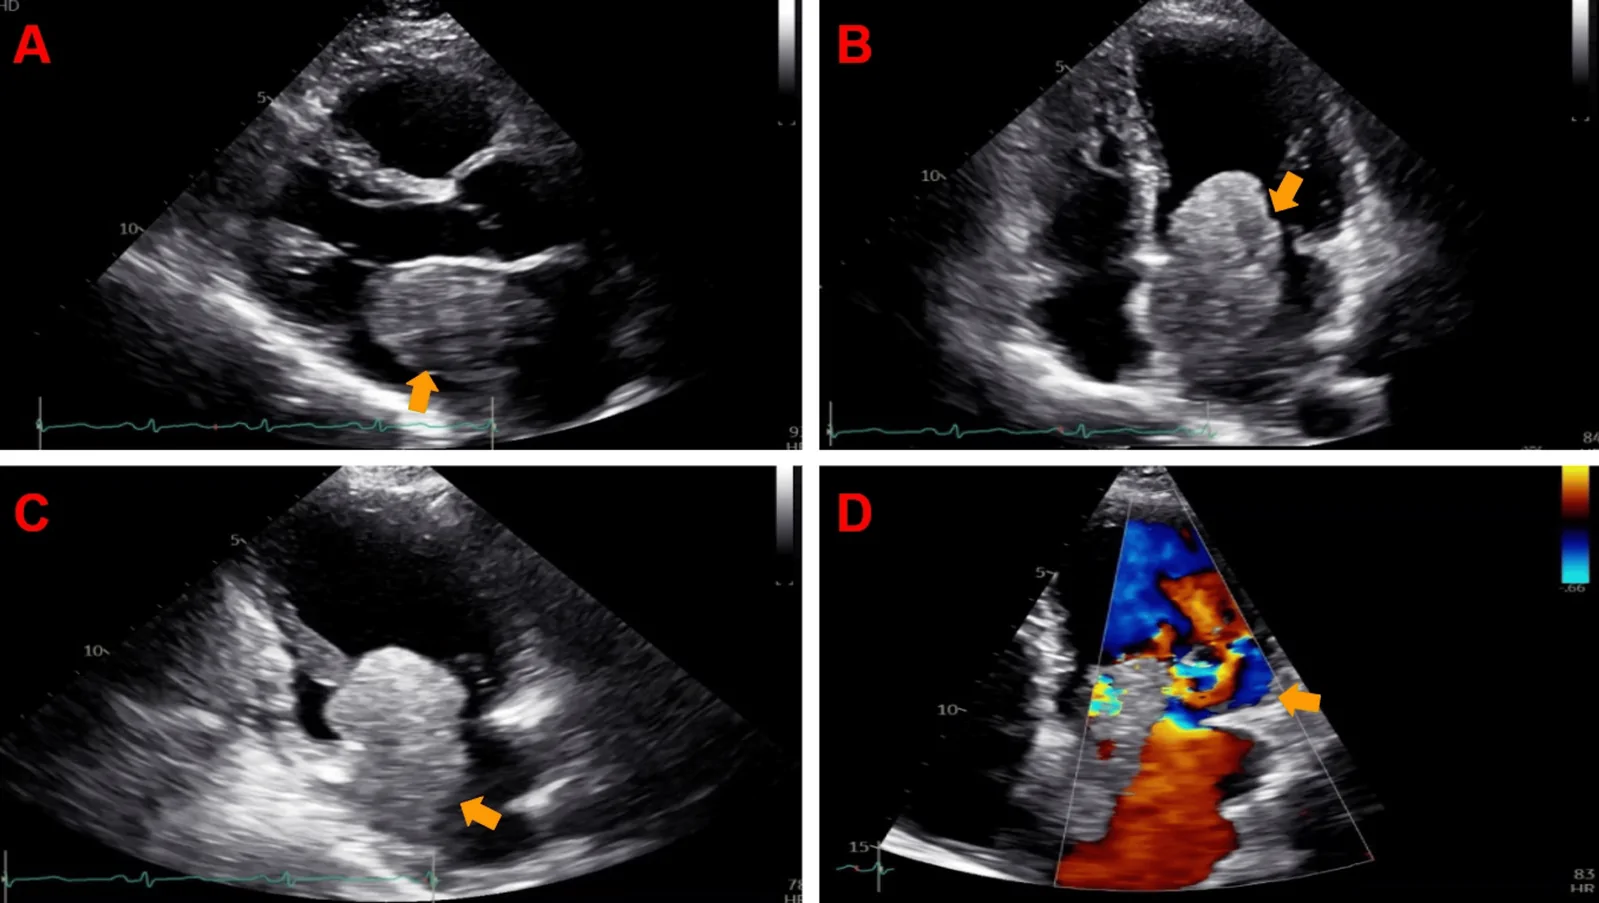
Transthoracic echocardiographic assessment of a giant left atrial myxoma (A) Parasternal long-axis view demonstrating a large, heterogeneous, highly mobile left atrial mass (orange arrow). (B) Apical four-chamber view showing diastolic prolapse of the mass (orange arrow) through the mitral valve into the left ventricle. (C) Apical four-chamber view demonstrating the pedunculated mass (orange arrow) attached to the interatrial septum, occupying nearly half of the left atrial cavity. (D) Color Doppler imaging demonstrating dynamic transmitral flow obstruction caused by the prolapsing mass (orange arrow), producing functional mitral stenosis through a "ball-valve" mechanism.
